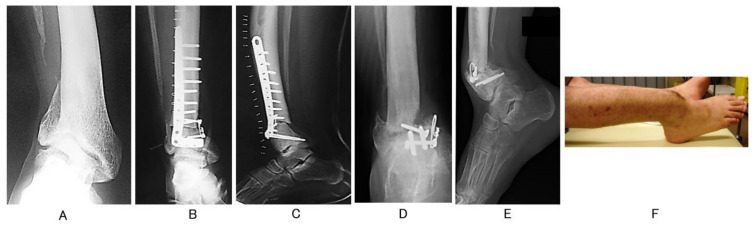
Clinical course prior to referral. (A) Preoperative anteroposterior radiograph showing ankle deformity associated with congenital fibular deficiency. (B,C) Anteroposterior and lateral radiographs immediately after deformity correction osteotomy performed at the previous institution. (D,E) Anteroposterior and lateral radiographs demonstrating nonunion at the osteotomy site with broken screws. (F) Clinical photograph at presentation showing anterior angulation at the nonunion site.
